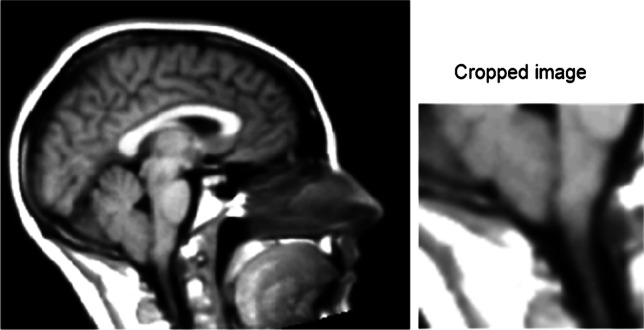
Example of a Chiari MRI misclassified as normal
